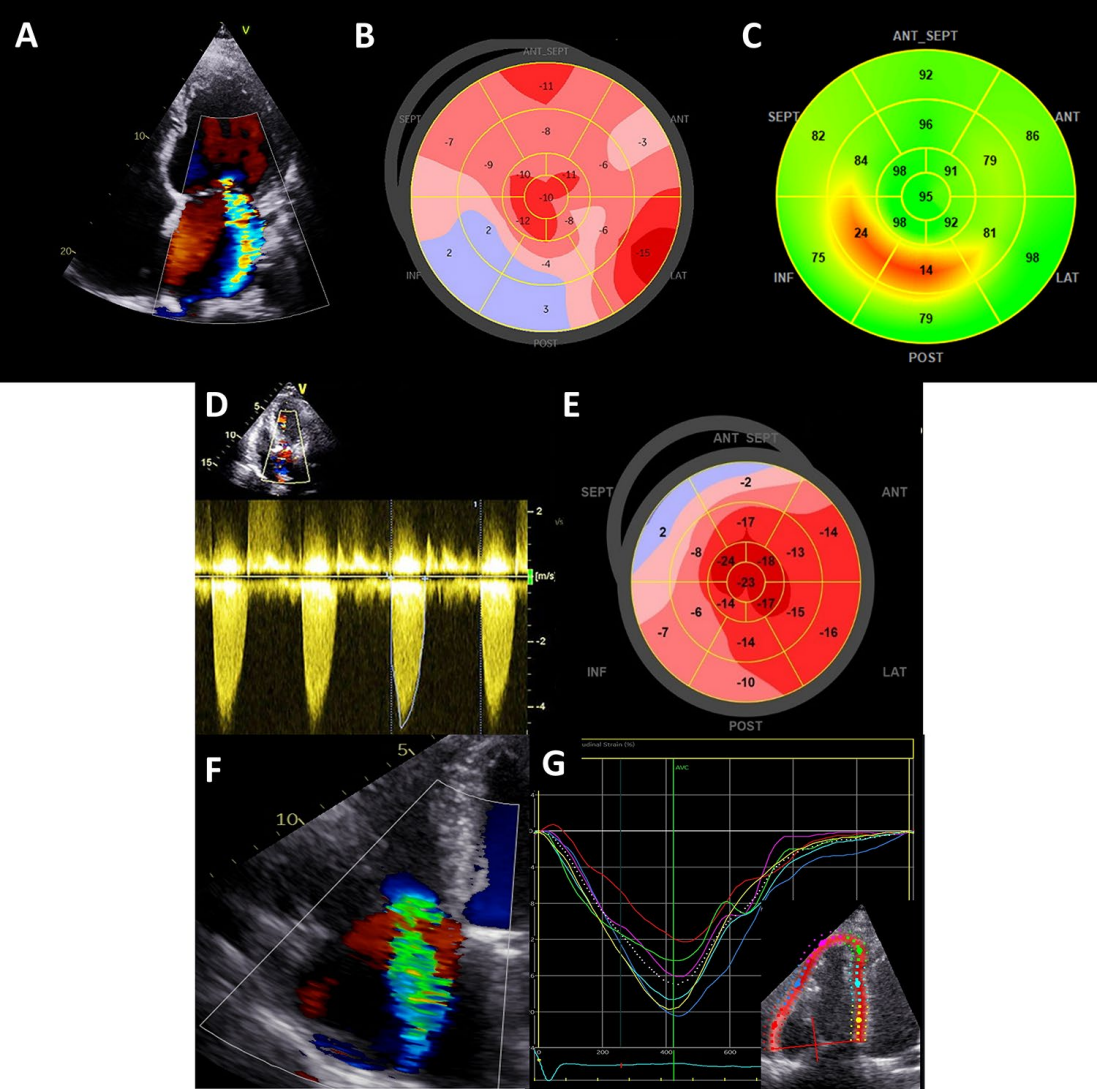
Speckle-tracking strain imaging in patients with valvular heart disease. The figure shows three examples of patients with valvular heart disease and the use of 2D speckle-tracking strain echocardiography. Patient 1 (panels A, B, C): a 67-year-old male patient with ischemic cardiomyopathy and non-viable myocardium after right coronary artery (RCA) myocardial infarction, presented with severe secondary mitral regurgitation due to tethering of the posteromedial papillary muscle (panel A). Left ventricular global longitudinal strain (GLS) was − 7.5%, with dyskinesia (blue color) in the RCA territory on a parametric GLS map (panel B). Impaired myocardial work efficiency can be seen in the RCA territory on a parametric map (panel C). Patient 2 (panels D, E): a 75-year-old male patient presented with severe aortic stenosis (aortic valve area 0.75 cm.^2^, peak aortic jet velocity 4.25 m/s, mean gradient 51 mmHg) (panel D). Although the left ventricular ejection fraction was preserved (56%), the left ventricular GLS was reduced (− 13.5%) (panel E). Patient 3 (panels F, G): a 69-year-old female patient presented with severe, secondary TR. Although tricuspid annular plane systolic excursion was preserved (21 mm) (panel F), right ventricular free wall strain was reduced (− 16%) (panel G)
